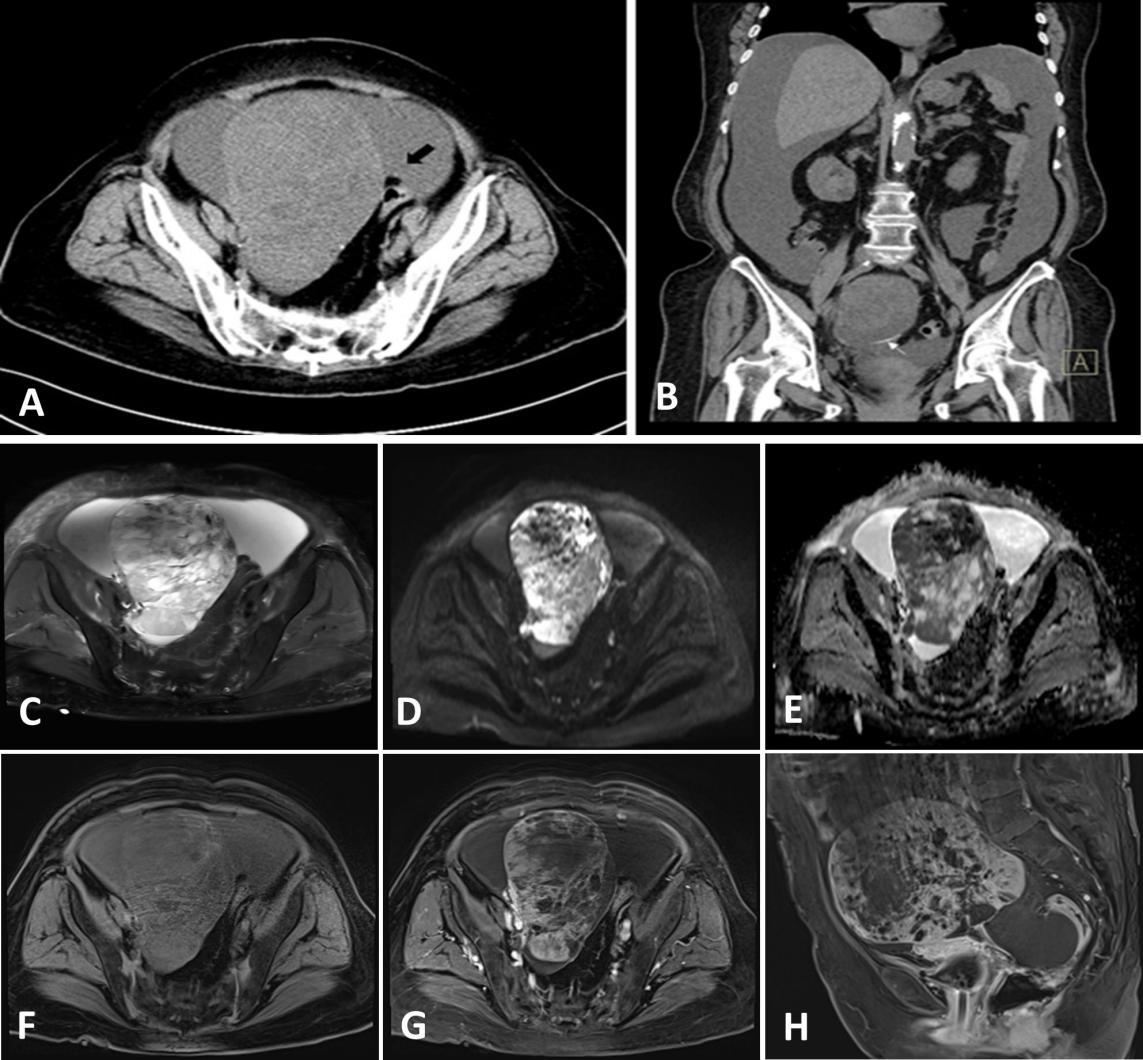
Computed tomography images (A, B) and enhanced magnetic resonance images (C–H) of the case.
